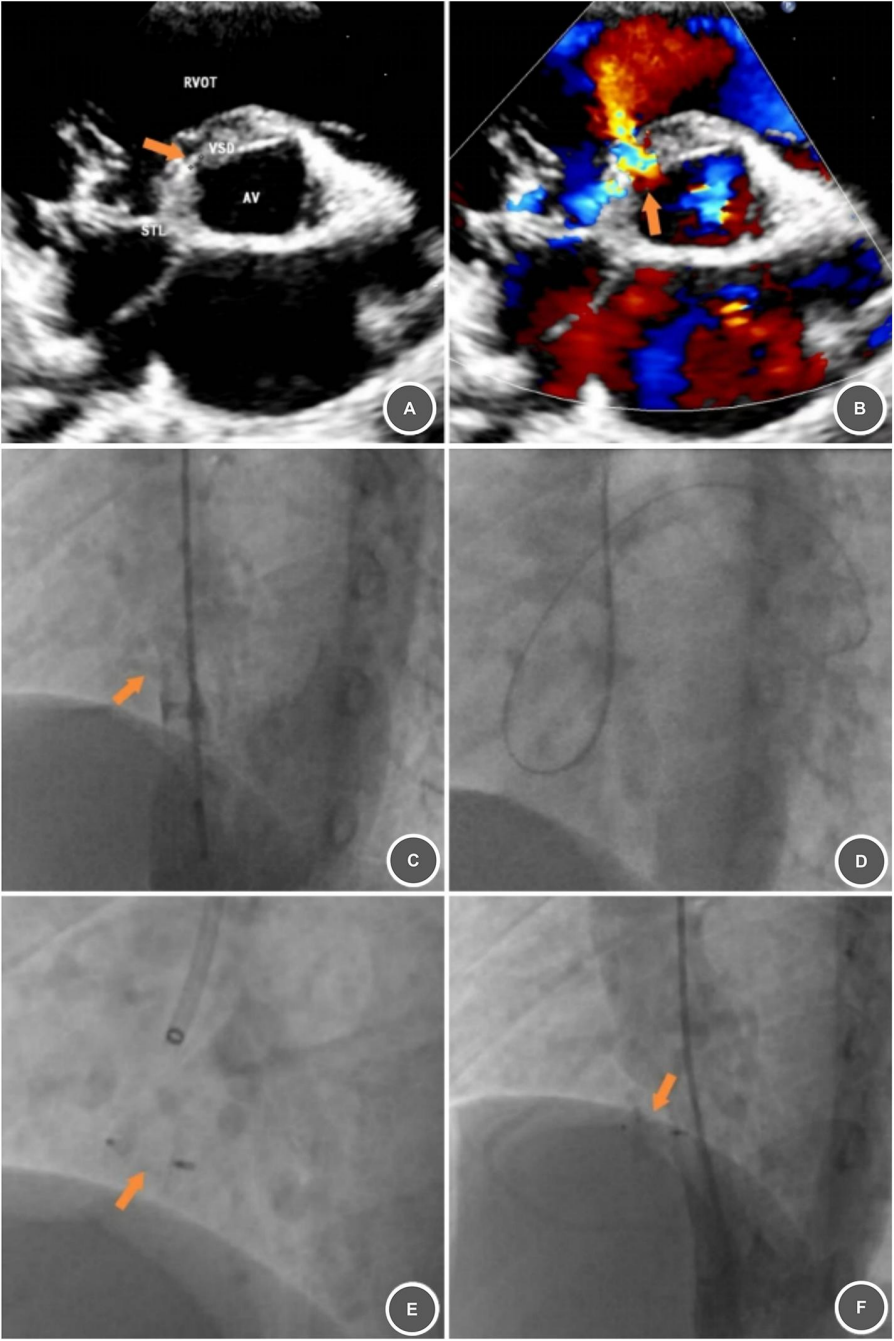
Preoperative and intraoperative imaging of a second perimembranous VSD case treated with a Starway Neo Occluder. (A,B) Preoperative echocardiography demonstrating a membranous ventricular septal defect (∼2.8 mm) with a visible color trans-septal jet (arrow). (C) Left ventricular angiography confirming VSD location and size (∼3.0 mm, arrow). (D) Established treatment tract. (E) Deployed Starway Neo Occluder (arrow). (F) Post-deployment angiography showing stable occluder position and morphology (arrow).
